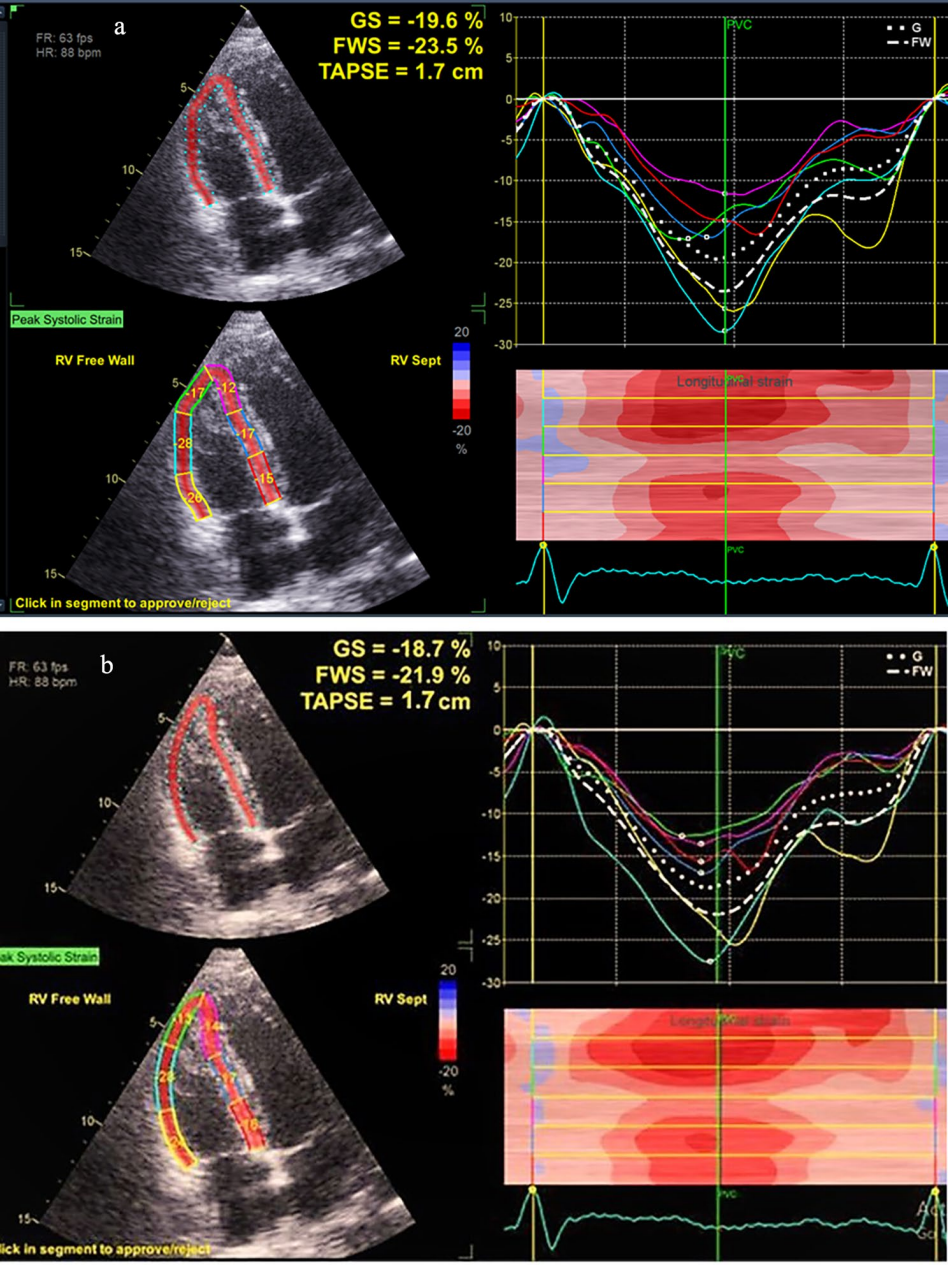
Tracking of the 6 – segment region of interest (ROI) in the endocardial border of the right ventricle (RV) from the apical four–chamber view was done. Then, RV free wall longitudinal strain(RVFWLS) was calculated by averaging peak systolic strain values of the three segments of the free wall, global longitudinal strain (GLS) was measured from the average values of peak systolic strain of RV free-wall and septal wall at (a) after 6 months and (b) after 9 months of chemotherapy
